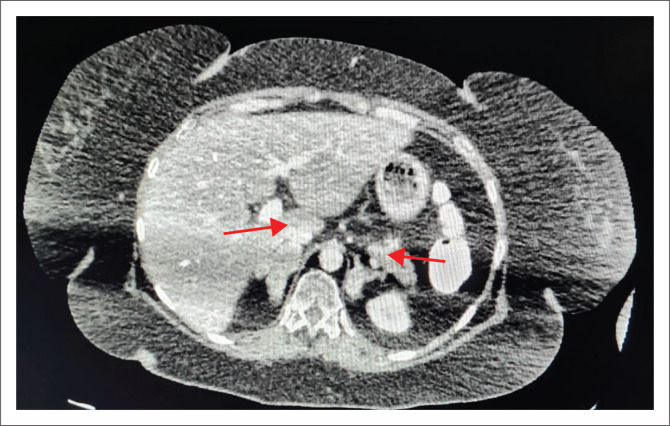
Computed tomography (axial view) demonstrating bilateral adrenal hyperplasia (arrows).
